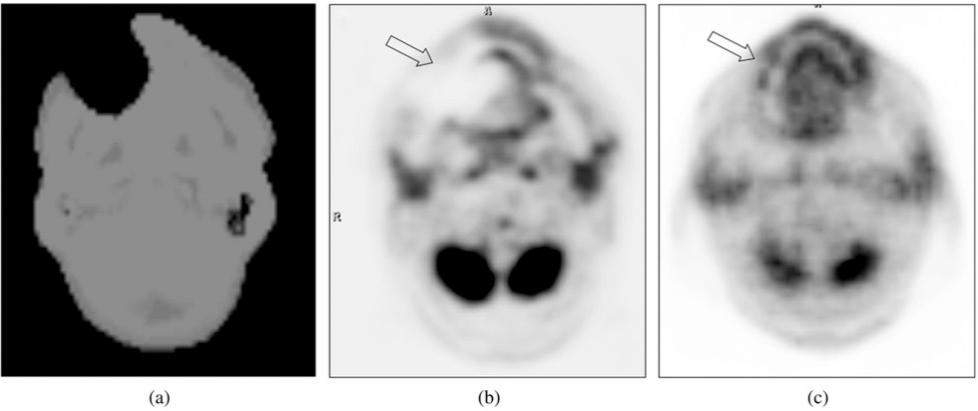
The MR-based attenuation image showing a typical artifact caused by a dental implant. (a) Shows a large region of missing data and tissue misclassification as air around the dental implants, resulting in an artificially decreased ^18^F-FDG uptake (arrow) in the corresponding MRAC PET (b) relative to the CT-based attenuation-corrected PET (c). Figure adapted from Buchbender et al. “Positron emission tomography (PET) attenuation correction artefacts in PET/CT and PET/MRI”, *Brit J Radiol* 2013, 27:1–9^13^ and reused with permission from the publisher, BIR.
